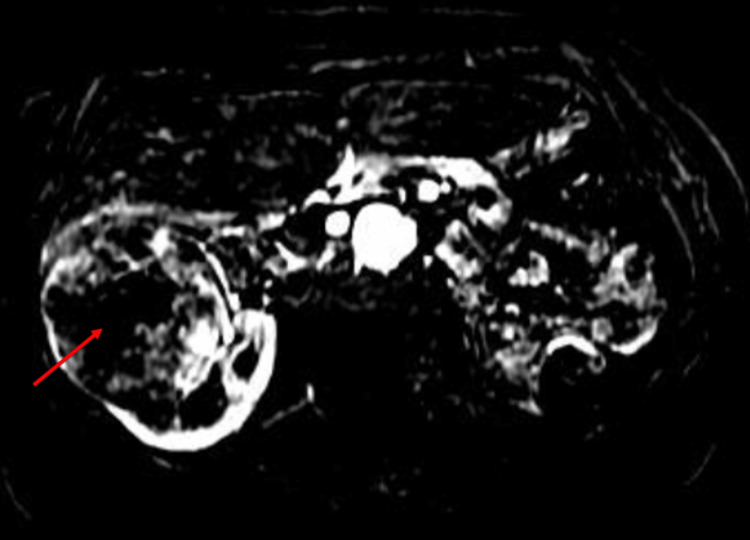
Right renal angiomyolipoma on MRI (red arrow).
